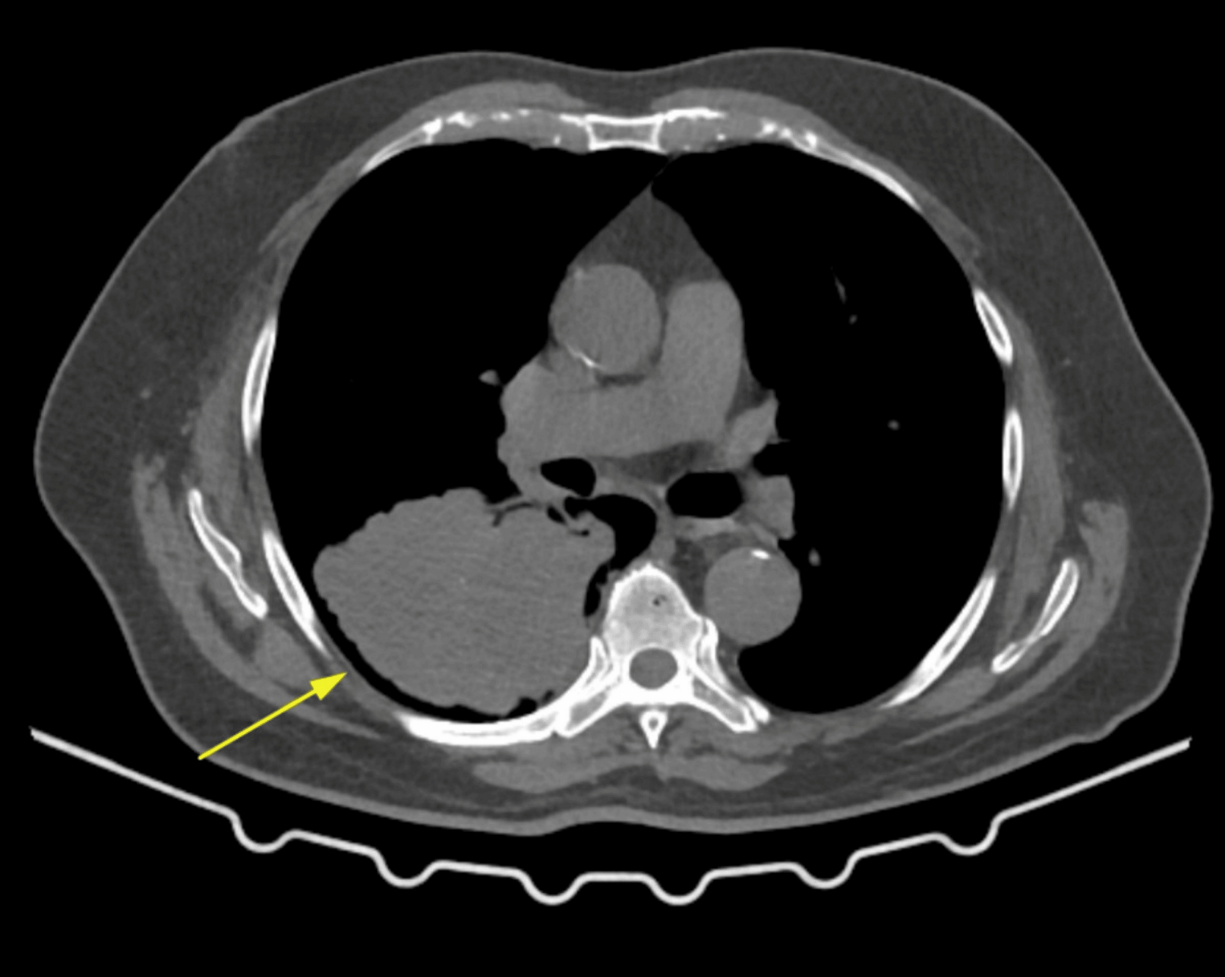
Contrast-enhanced CT of the chest showing a heterogenous lobulated mass in the right lower lobe of the lung (arrow) CT: computed tomography
